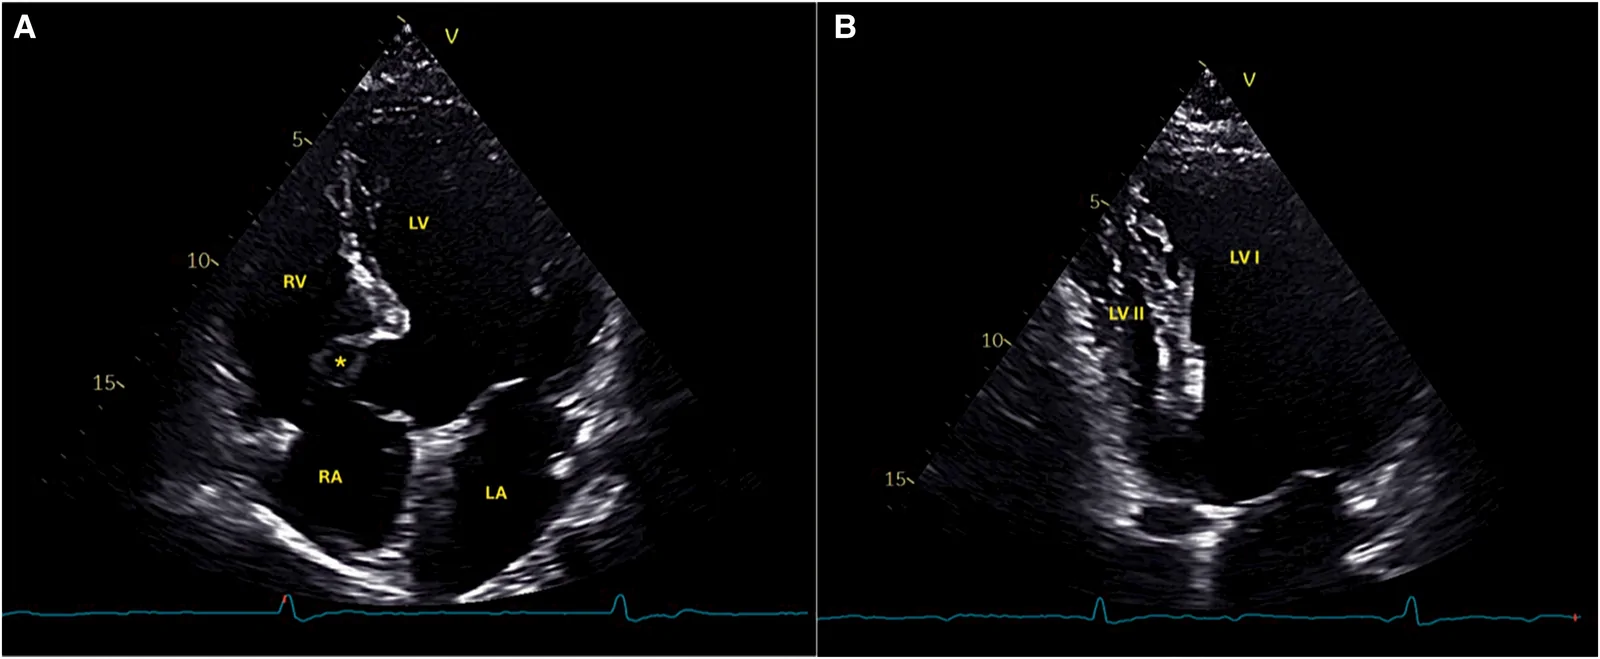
Transthoracic echocardiographic images showing a perimembranous interventricular septal aneurysm (marked with asterisk) and a left ventricular accessory chamber (LV II). (*A*) Two-dimensional TTE apical four-chamber view. (*B*) Apical two-chamber view. LA, left atrium; LV/LV I, left ventricle/primary chamber of the left ventricle; LV II, accessory chamber of the left ventricle; RA, right atrium; RV, right ventricle.
